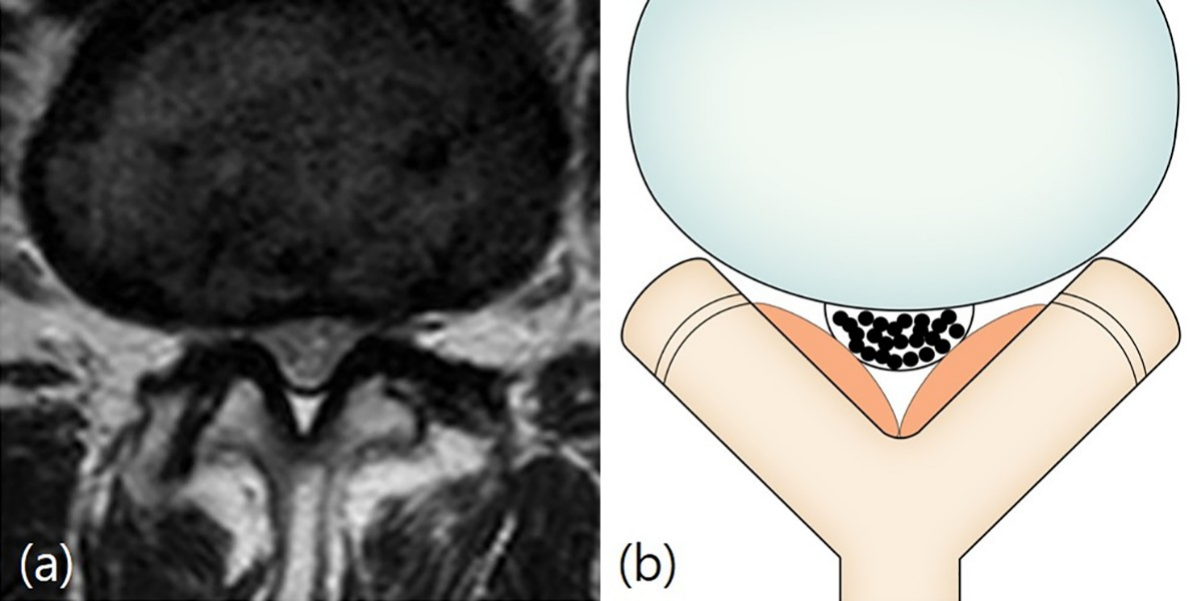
Lee classification. Grade 2 (moderate stenosis). Fig 5A and 5B: A 59-year-old woman with right lower extremity radiculopathy. T2-weighted axial magnetic resonance image at L4/5 disc level shows moderate obliteration of the anterior cerebrospinal fluid space and some cauda equina aggregation.
